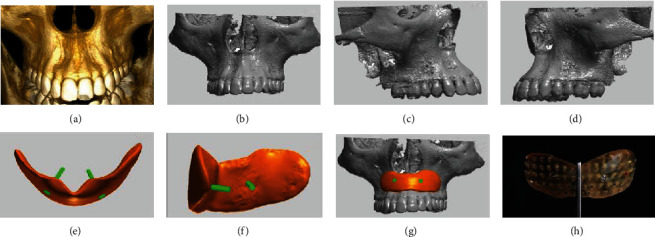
(a) Cone-beam computed tomography scan was performed to evaluate the subnasal depression and to plan the PMMA block. (b–g) Planning of the PMMA block in a 3D planning software according to the defect geometry. (h) Printed PMMA block.
